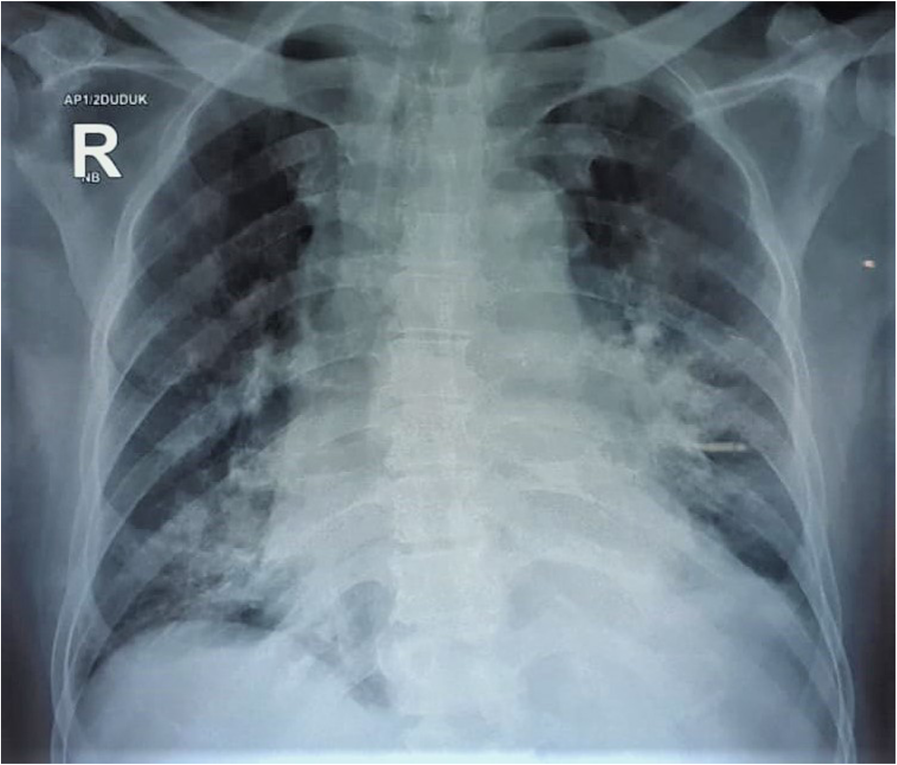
Chest X-ray showed preceding consolidation persisted with new consolidative changes in the left apical-middle-lower zone and the right lower peripheral region
